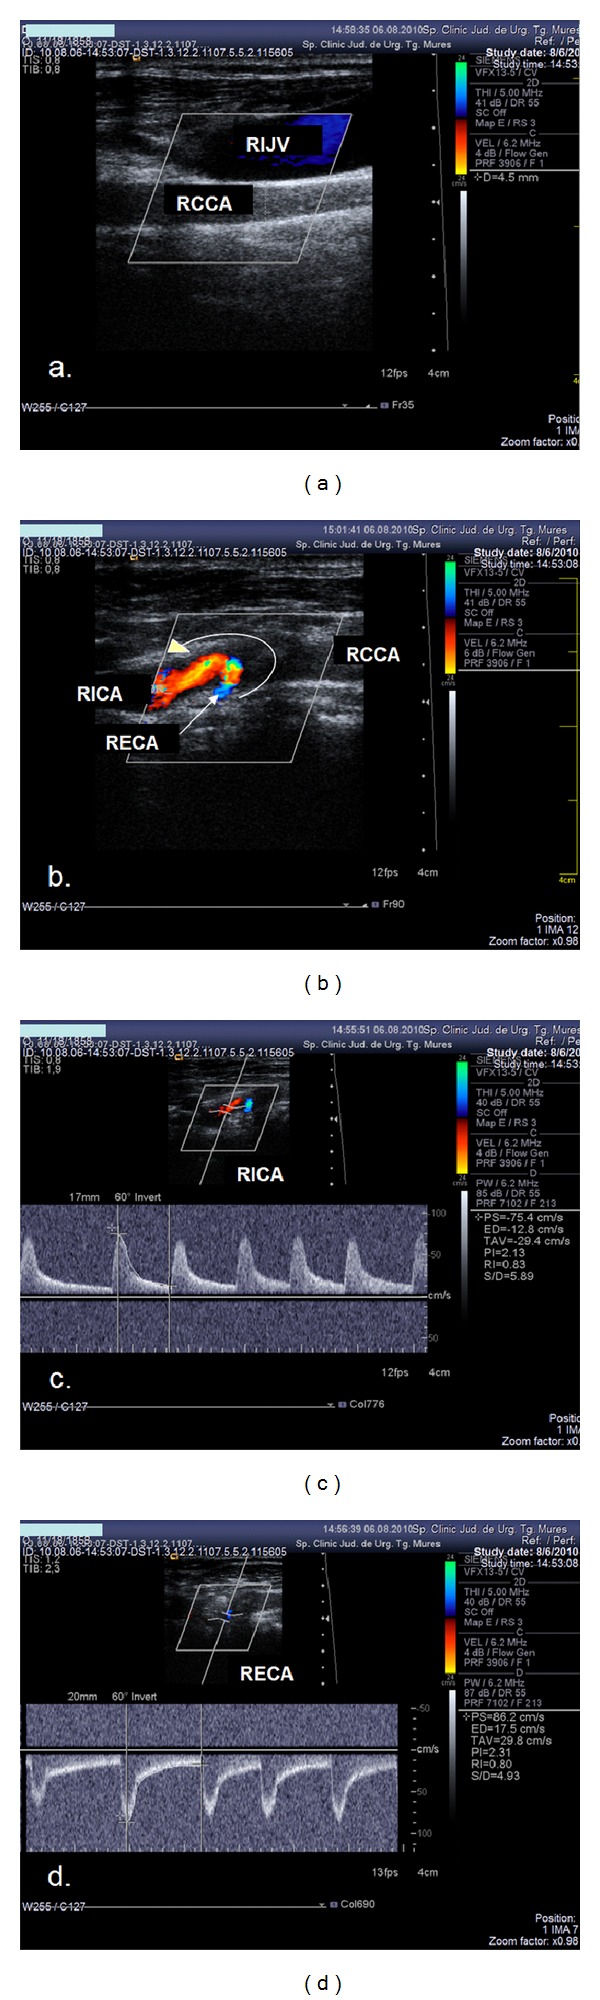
Ultrasound examination showing CCA occlusion with patent distal vessels. (a) Colour mode examination: no flow in the right common carotid artery, and the vessel lumen is filled with thrombotic material. (b) Colour mode examination of carotid bifurcation: reversed flow in the ECA, anterograde flow in the ICA. (c) Duplex mode examination: anterograde flow in the ICA. (d) Duplex mode examination: retrograde flow in the ECA.
